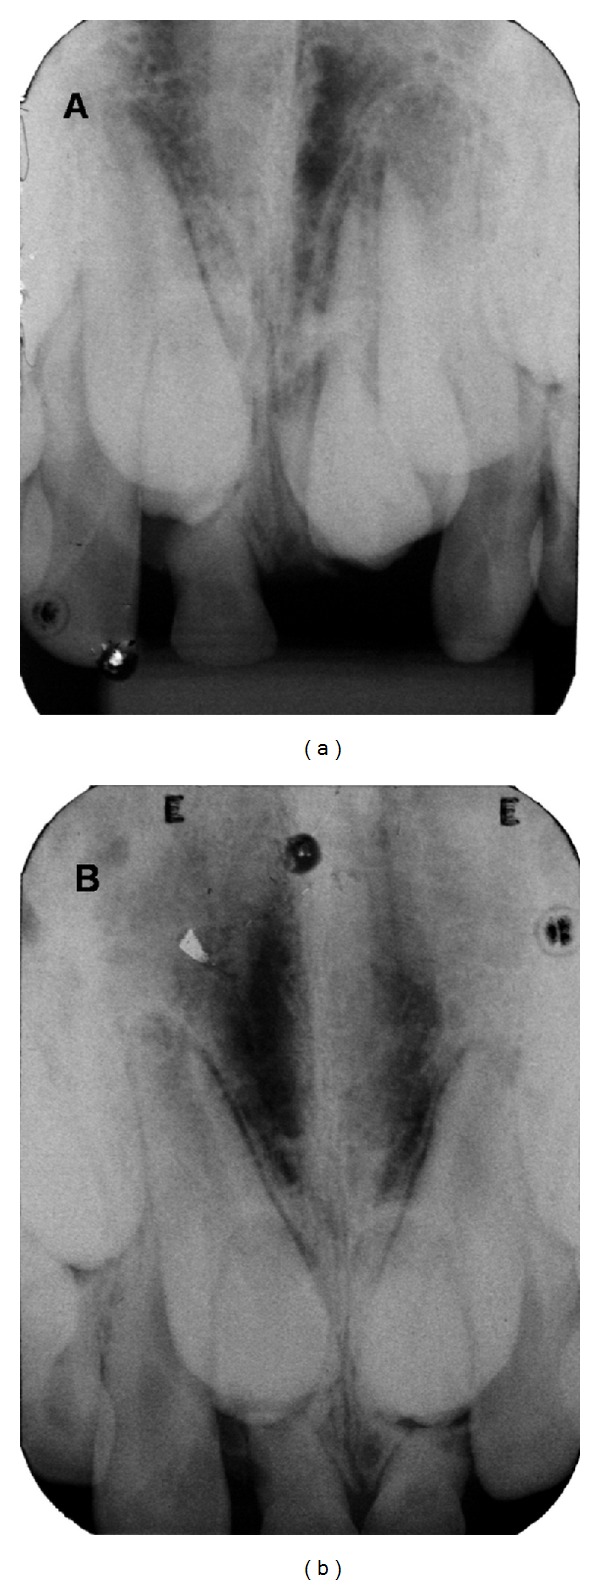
Periapical radiograph showing impacted permanent maxillary central incisors and the presence of two mesiodens in both twins.
